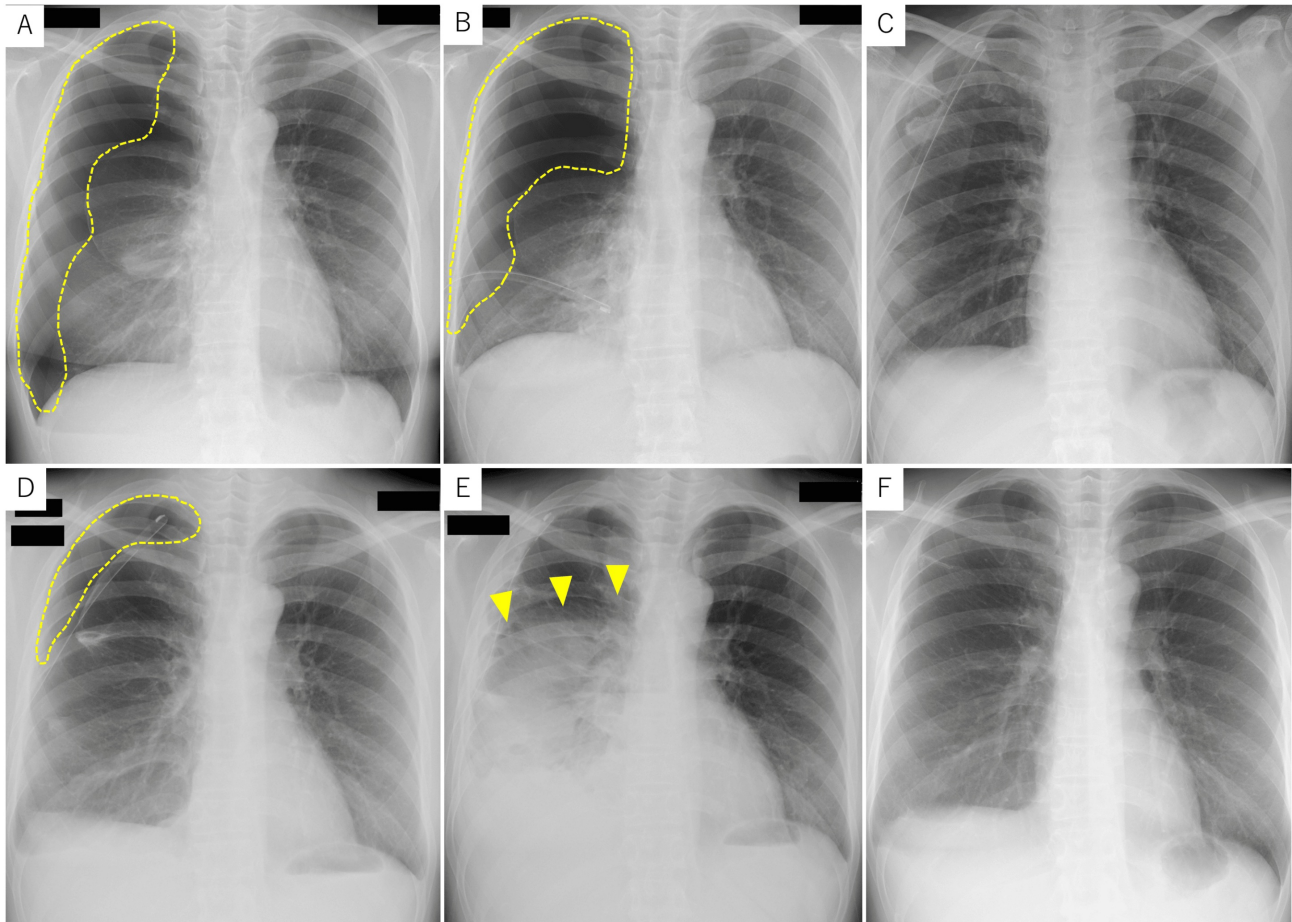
(A) Chest radiograph demonstrating right lung collapse consistent with pneumothorax (demarcated by a yellow dotted line). (B) Despite chest tube placement, the right lung collapse progressed (demarcated by a yellow dotted line). (C) Postoperative chest radiograph obtained on the following day demonstrated satisfactory lung expansion. (D) On postoperative day three, chest radiography revealed inadequate expansion of the right lung (demarcated by a yellow dotted line). (E) Postoperative day 13 chest radiograph revealed right pleural effusion (yellow arrowheads); however, aeration of the right lung had improved following three pleurodesis procedures. (F) Chest radiograph obtained 2.5 years post-thoracotomy for pneumothorax revealed no evidence of pulmonary collapse.
